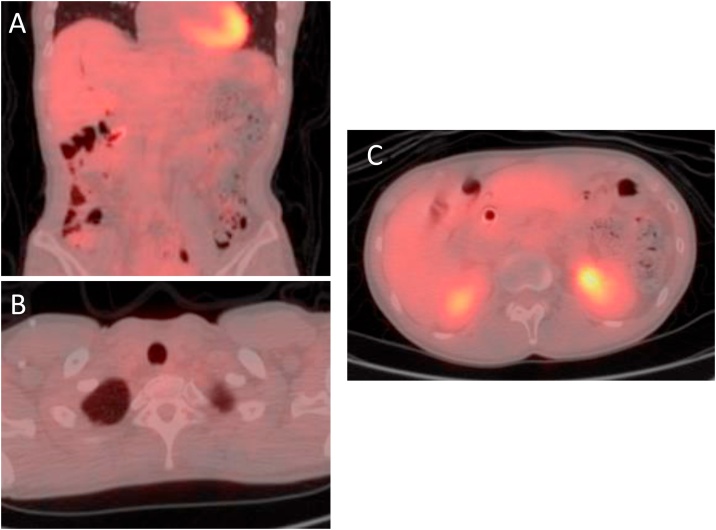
Positron emission tomography (PET)-CT scan just before conversion surgery showing no distant metastases. A: para-aortic lymph nodes; B: left supraclavicular lymph node; C: primary lesion.
